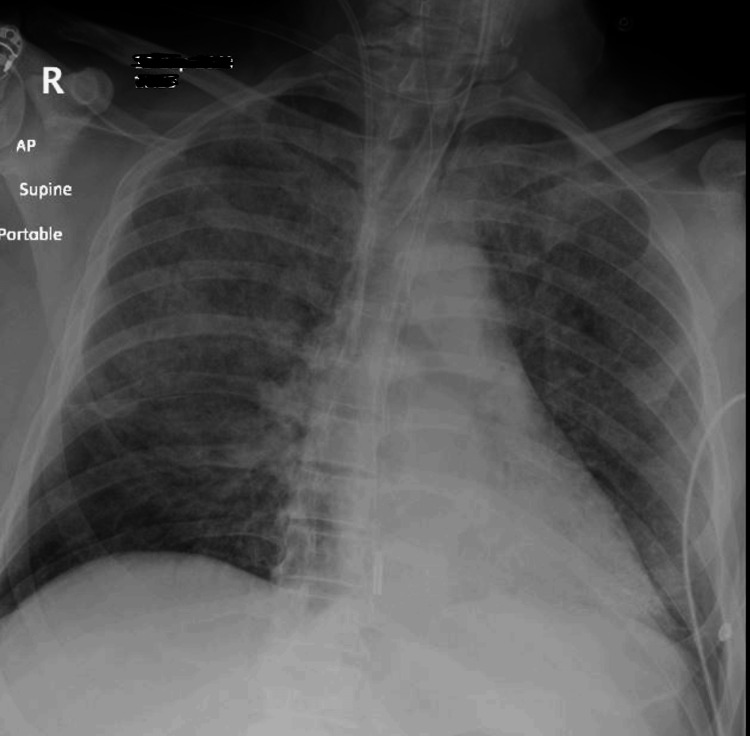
Portable chest X-ray in theatre showing bilateral lung opacifications
